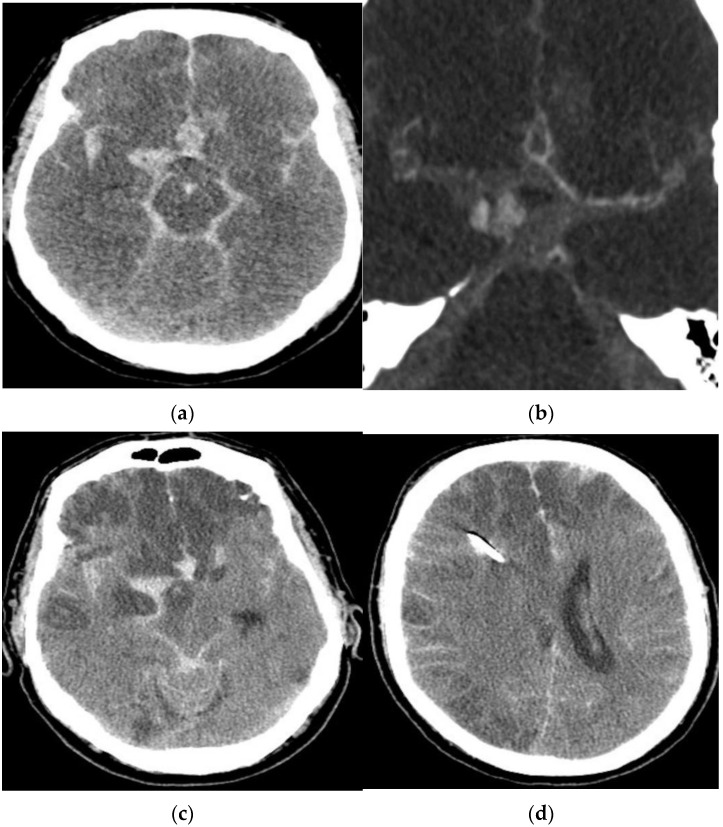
Diagnostic imaging of a 57-year-old male patient with an aSAH due to a ruptured MCA aneurysm. Computed tomography (a) and CTA (b) revealed a massive aSAH and early vasospasm. CCT after one day (c,d) showed large ischemic infarctions.
